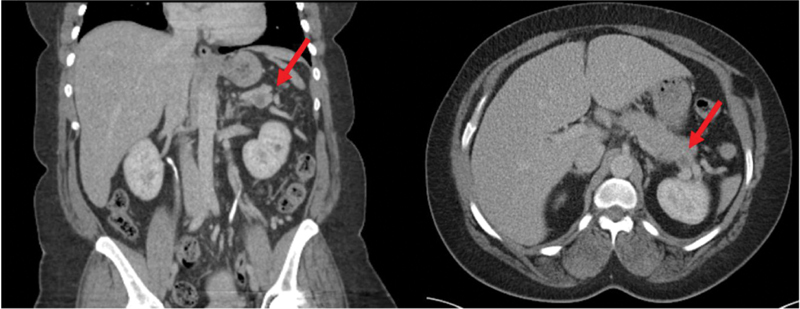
Pancreatic lesion (arrow) on computed tomography imaging.
